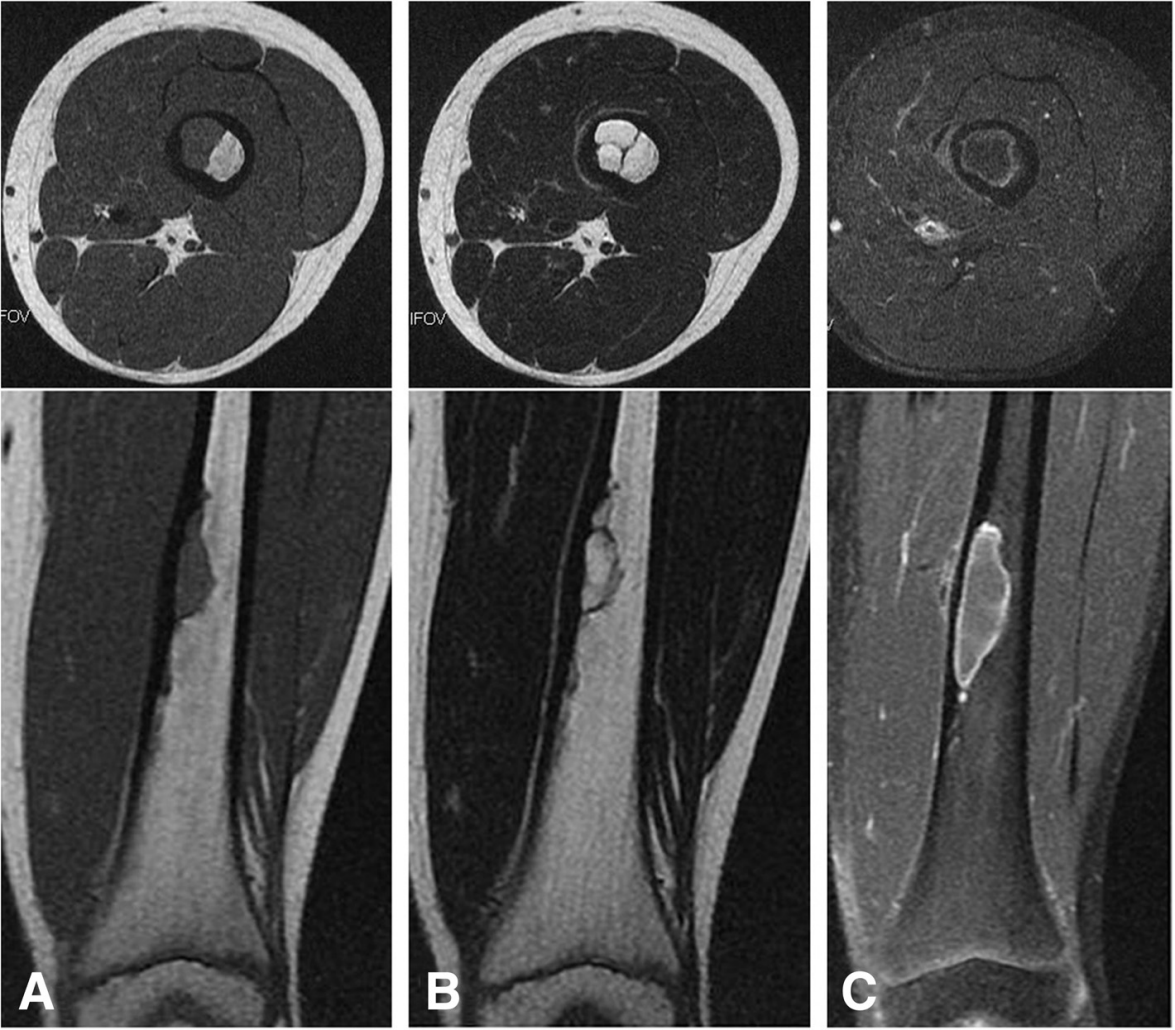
Magnetic resonance imaging shows a medullary lesion with low signal intensity on (A) T1- and (B) T2-weighted images. The size of the lesion increased over 10 months (C). Enhancement by gadolinium is seen on the periphery of the lesion on the T1-weighted image (C).
